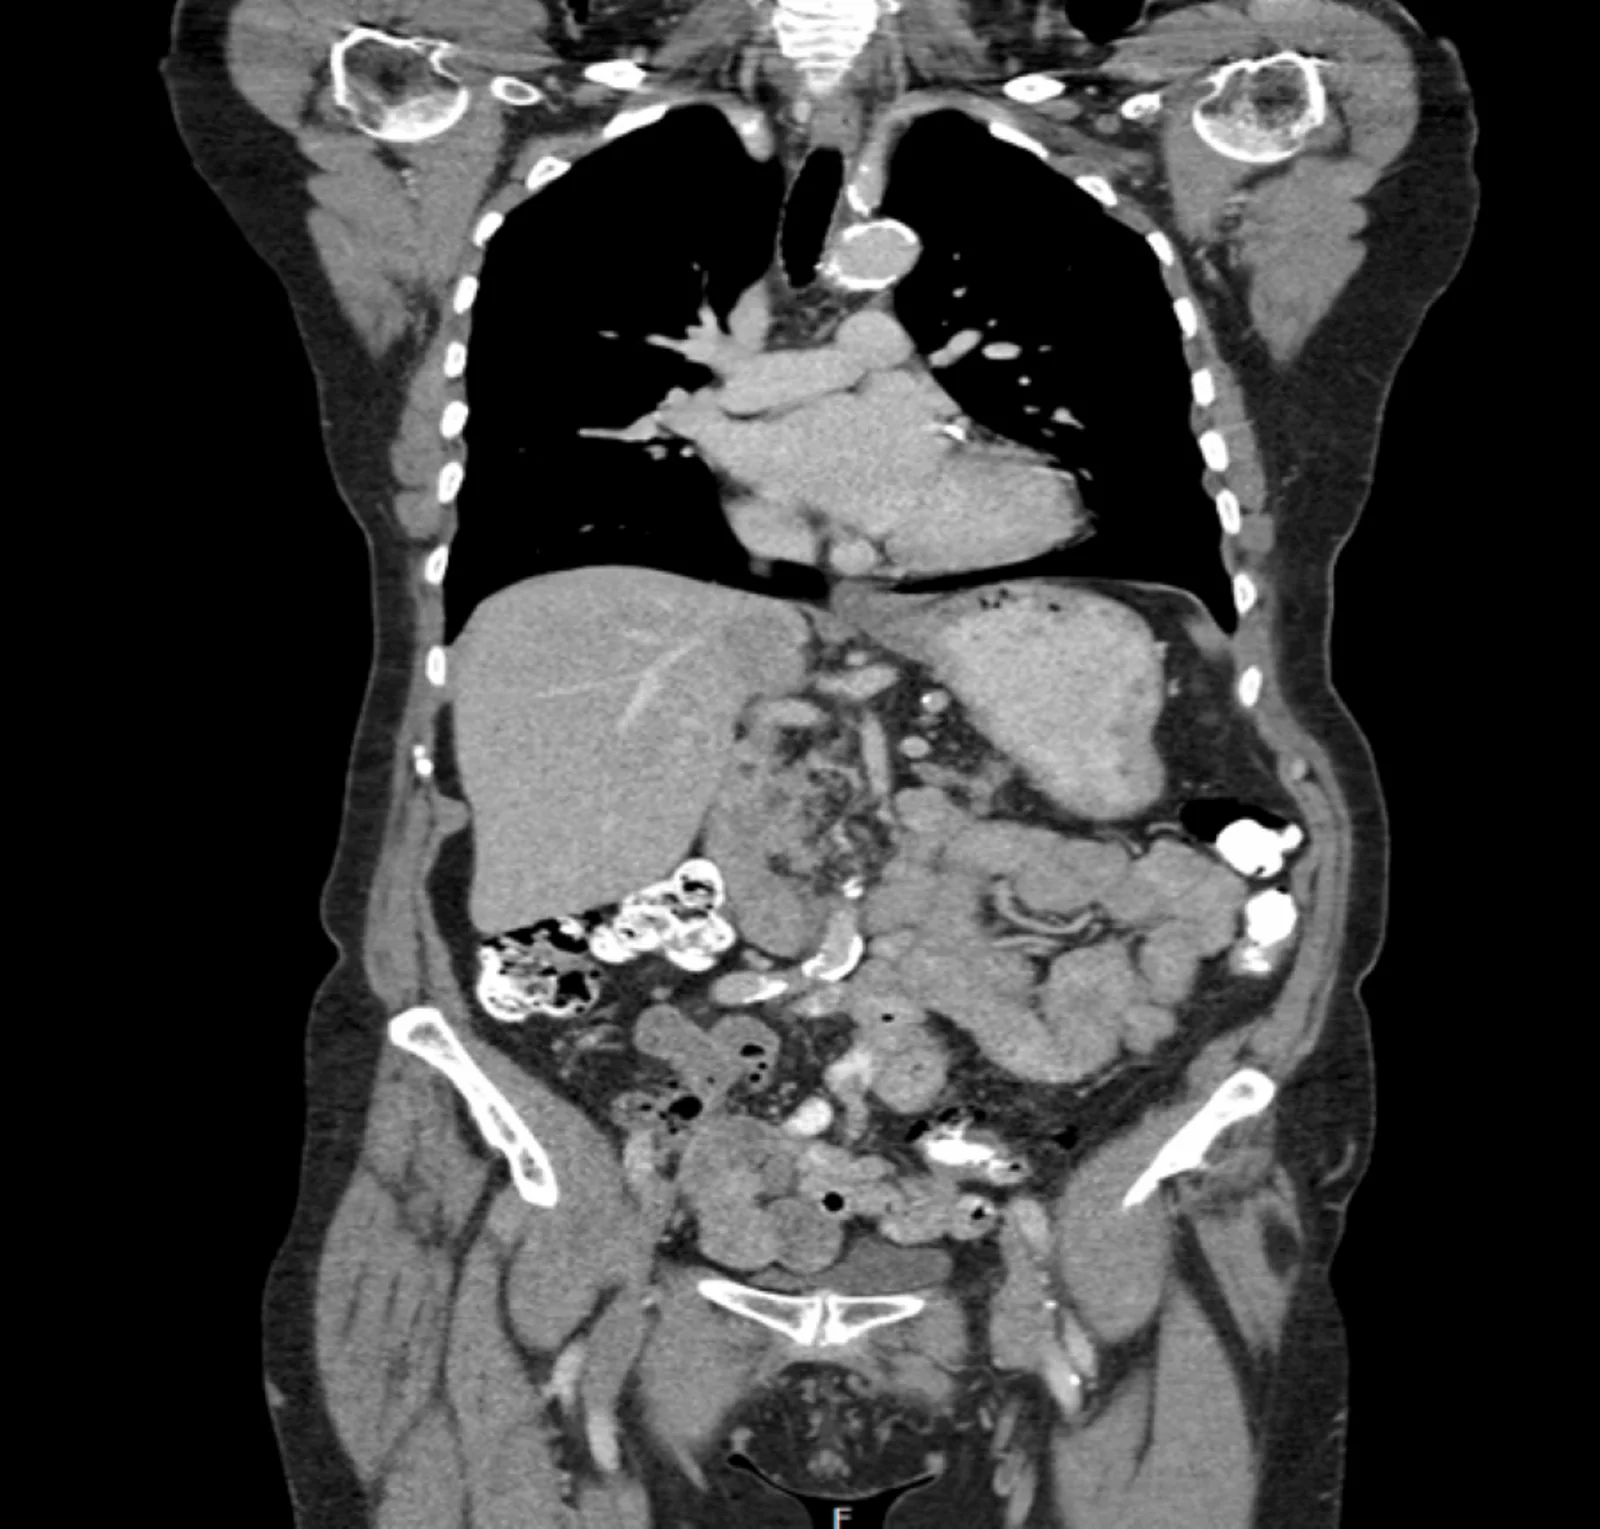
CT of the thorax, abdomen, and pelvis performed four months previously as part of the work-up for potential malignancy due to iron-deficiency anaemia was normal, with no evidence of malignancy or pancreatic pathology.
